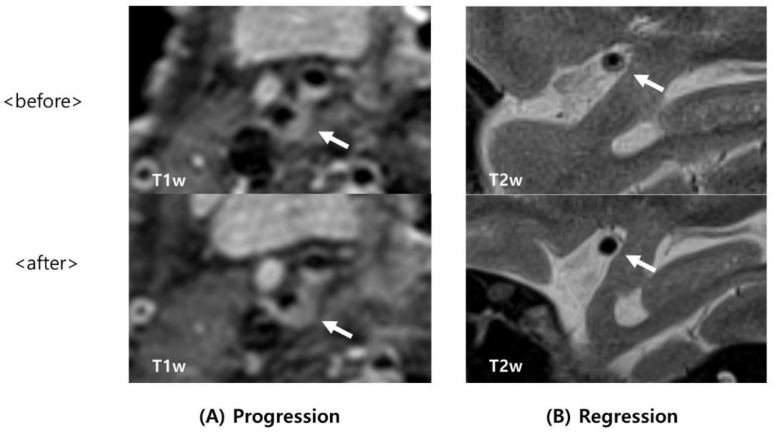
Examples of progression and regression of cerebral artery stenosis on HR-MRI. (A,B): Atherosclerotic plaque (arrow). (A) Upper panel: initial T1-weighted (T1w) HR-MR imaging reveals moderate stenosis in the right ICA; lower panel: follow-up T1w HR-MR imaging indicates severe stenosis in the right ICA. (B): Upper panel: initial T2-weighted (T2w) HR-MR imaging of the MCA with stenosis. Lower panel: follow-up T2w HR-MR imaging shows the MCA without stenosis. HR-MRI = high-resolution magnetic resonance imaging; ICA = internal carotid artery; MCA = middle cerebral artery.
